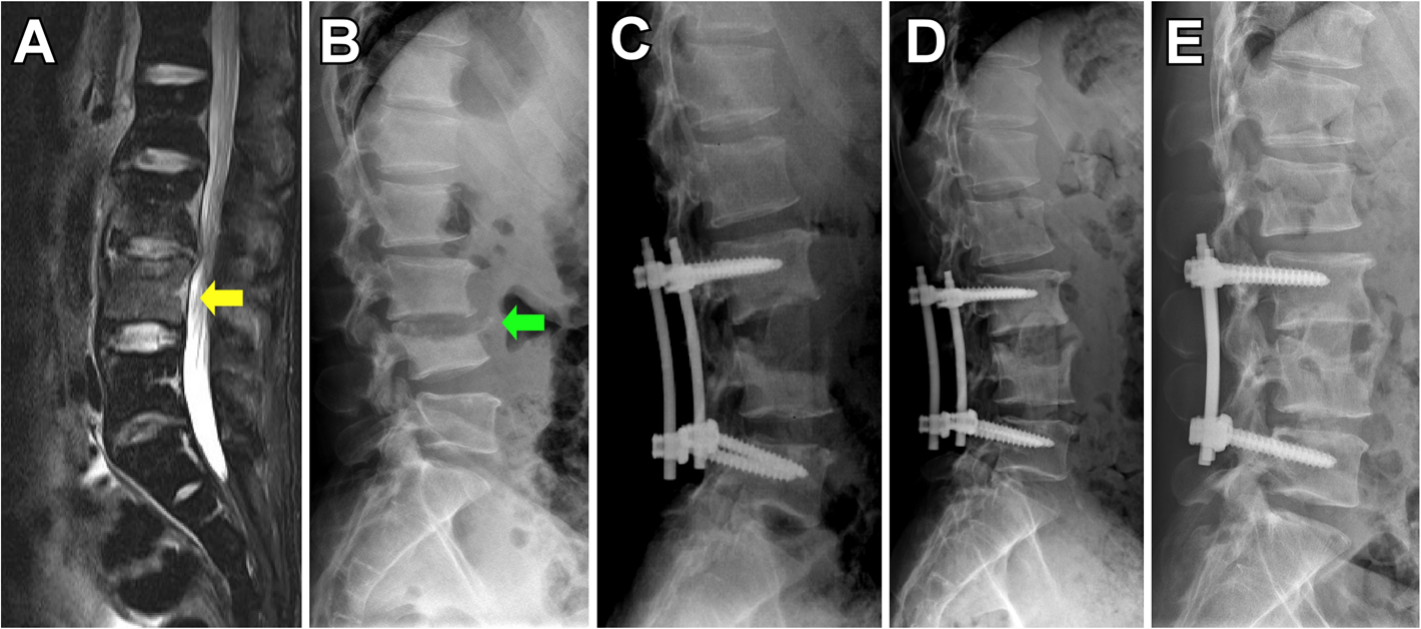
Pre- and post-treatment radiological images of a 45-year-old male with intractable low back pain and limited range of motion of waist. a, at first consultation, intensified signal at L3, L4 vertebral bodies were seen on T2-weighted MRI (yellow arrow). b, treated for 6 weeks with intravenous antibiotic therapy alone, the symptoms deteriorated and L4 body destructed (green arrow). c, instant X-ray filming after a combined surgical approach of posterior instrumentation and anterior debridement and fusion. d, postoperative X-ray filming at the 6-month follow up. E, postoperative X-ray filming at the 12-month follow up
